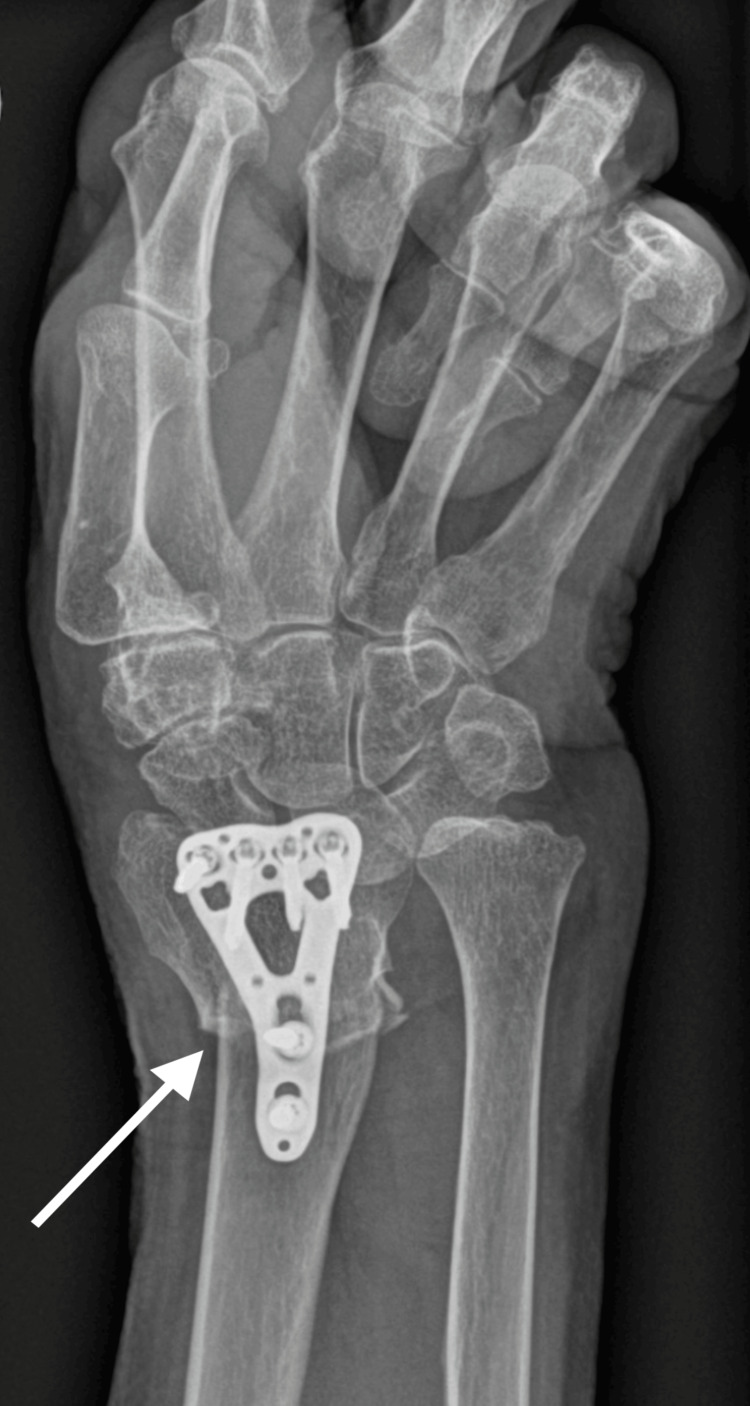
X-ray of radius refracture with bending plate, anterior posterior view
